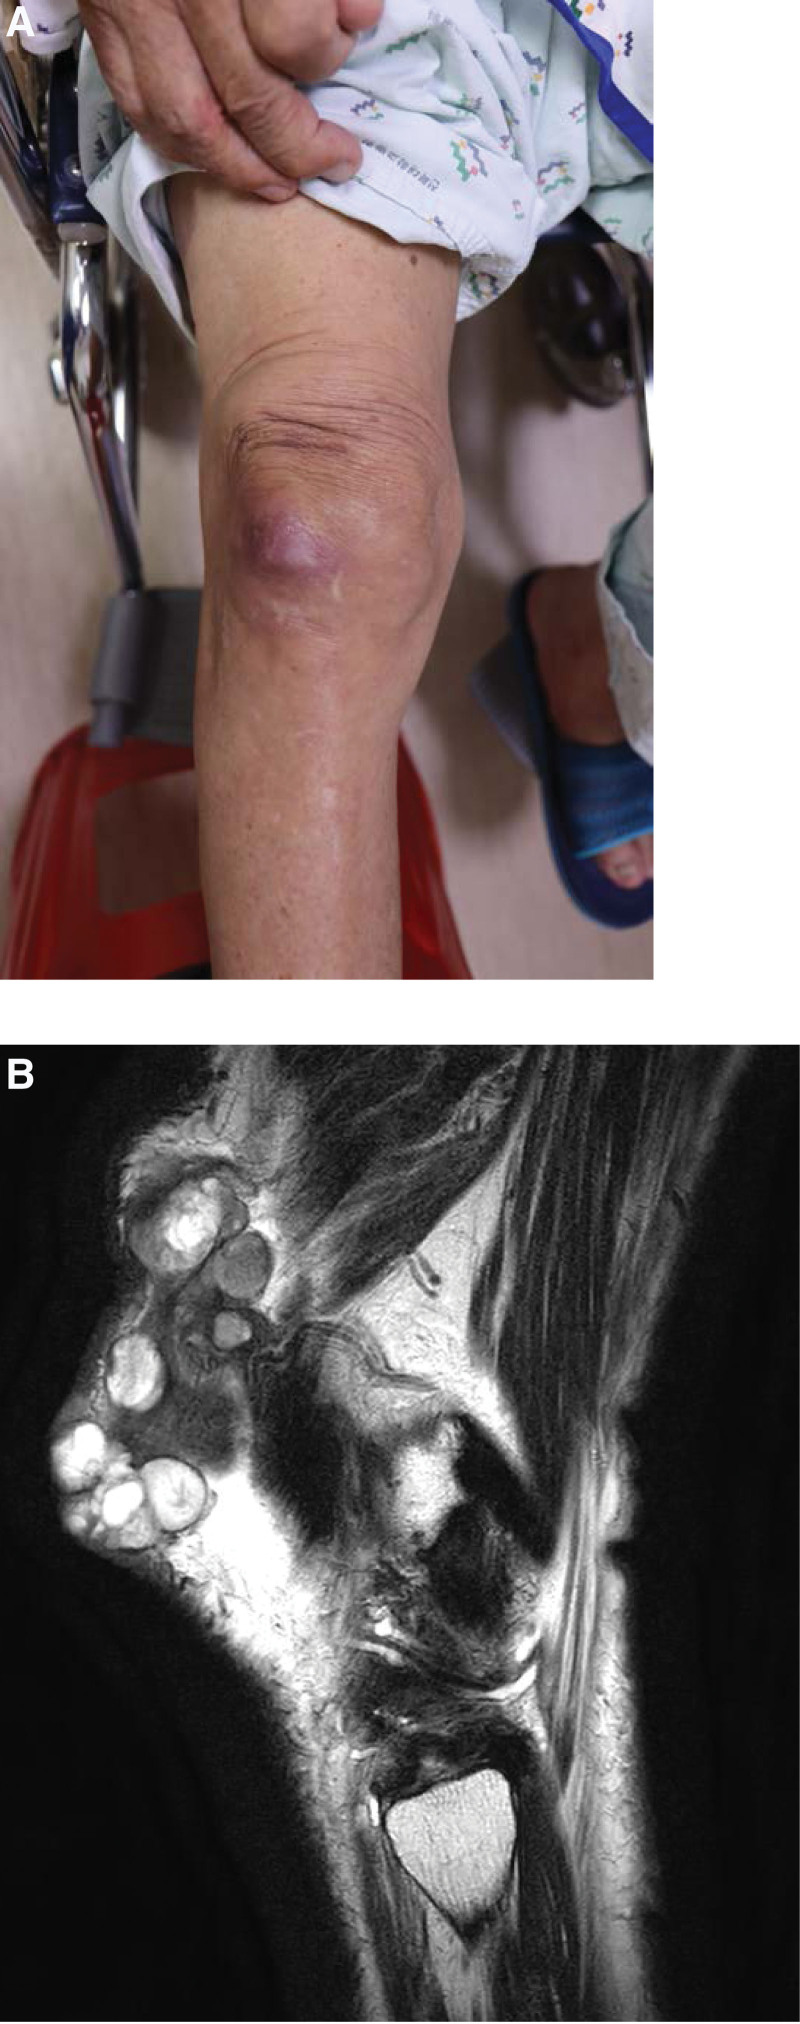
(A) About 12 × 10 cm sized mass was palpated on subcutaneous layer of right knee. (B) MRI view. MRI = magnetic resonance imaging.
